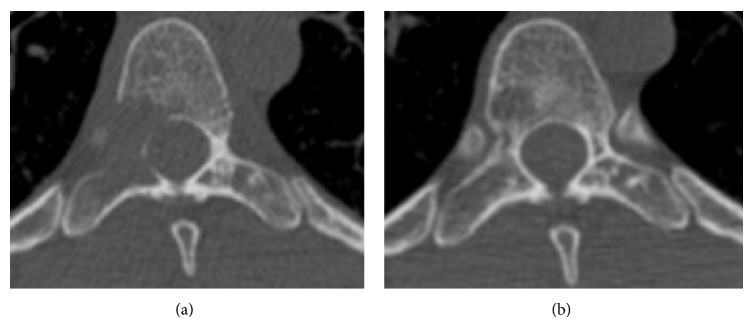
(a) Computed tomography of the spine showed a large solitary osteolytic lesion of thoracic number 8 vertebral body and right pedicle. (b) Repeat CT spine, 18 months later, showed evidence of significance bone regeneration at the T8 osteolytic lesion.
